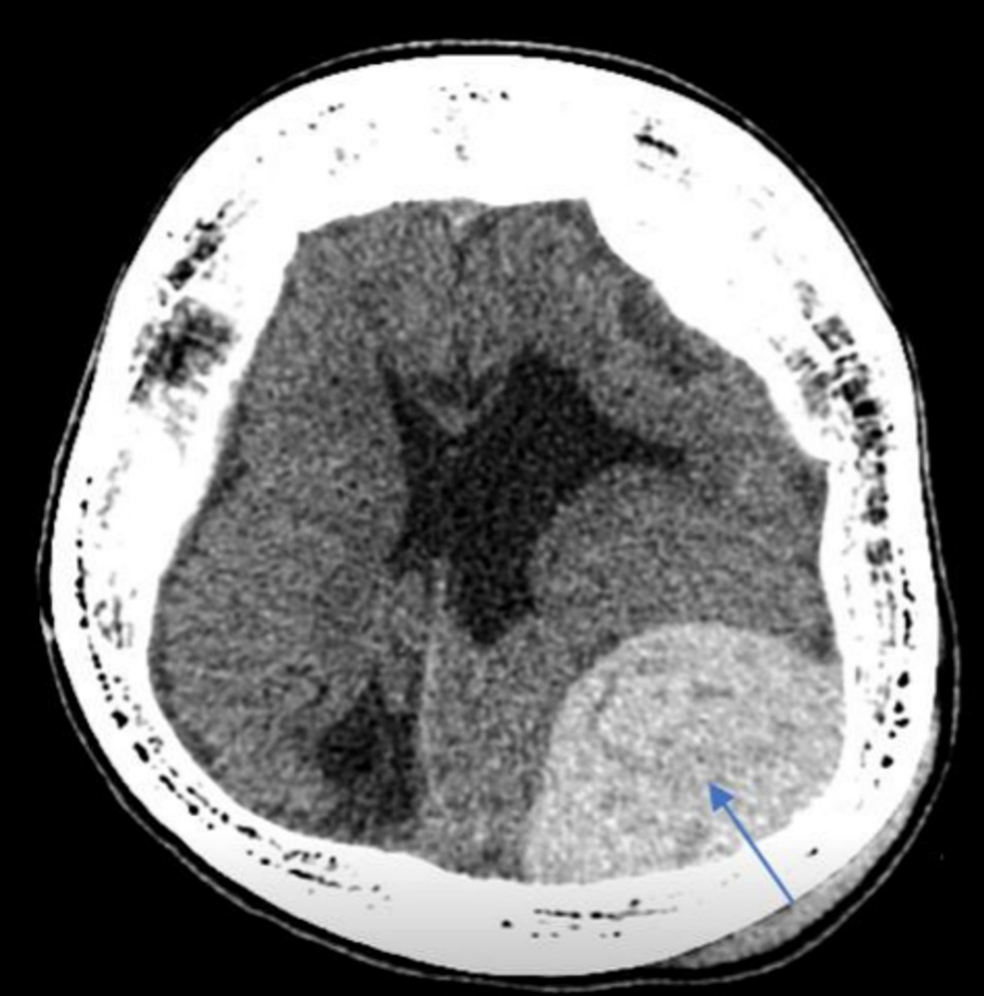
The plain CT brain showed acute left parietal epidural hematoma at the convexity of the left parietal bone (pointed in the image), measuring 9.7 x 5.2 x 8.4 cm in maximum AP, CC, and transverse dimensions with significant mass effect and a midline shift of 4 mm. AP: anterior-posterior, CC: craniocaudal
